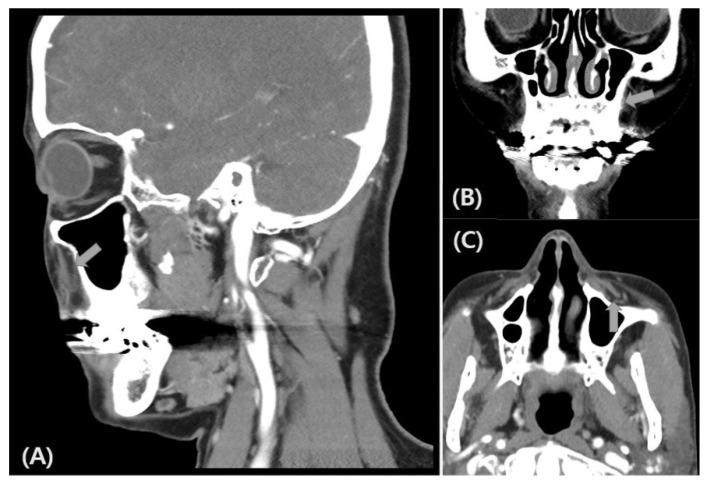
Sagittal (A), coronal (B) and axial (C) facial computed tomography images after implant removal. There is no implant at the nearby infraorbital nerve (arrows in A–C).
